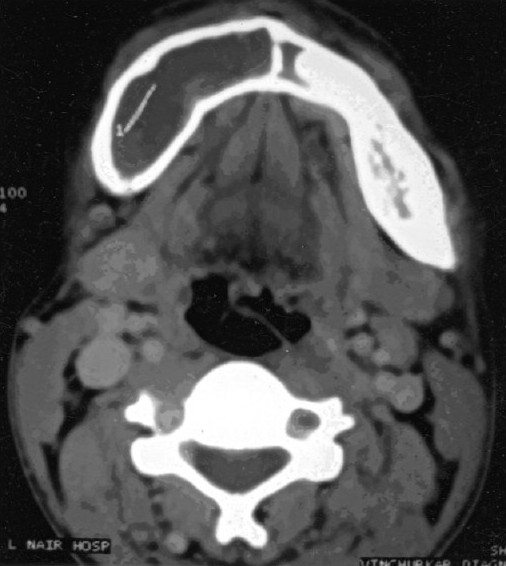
Computed tomogram of mandible showing large expansile lytic lesion in the right hemimandibular region
